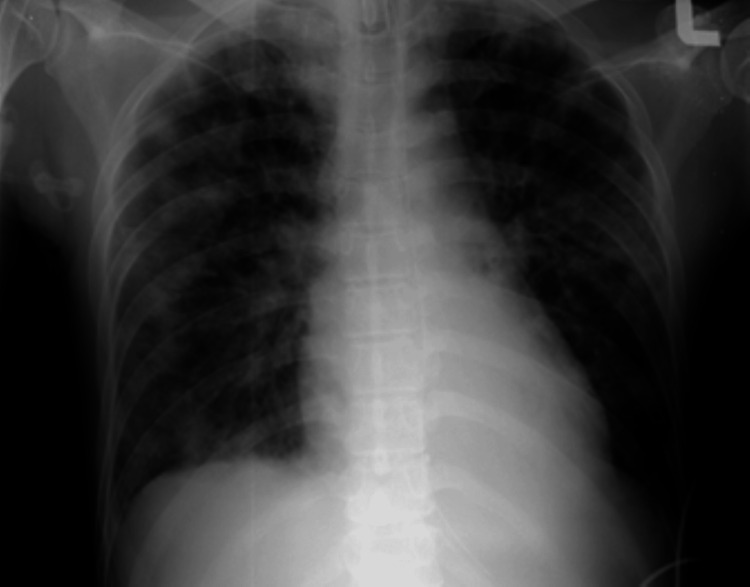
Chest X-ray showing generalized ground-glass opacities over both lung fields
